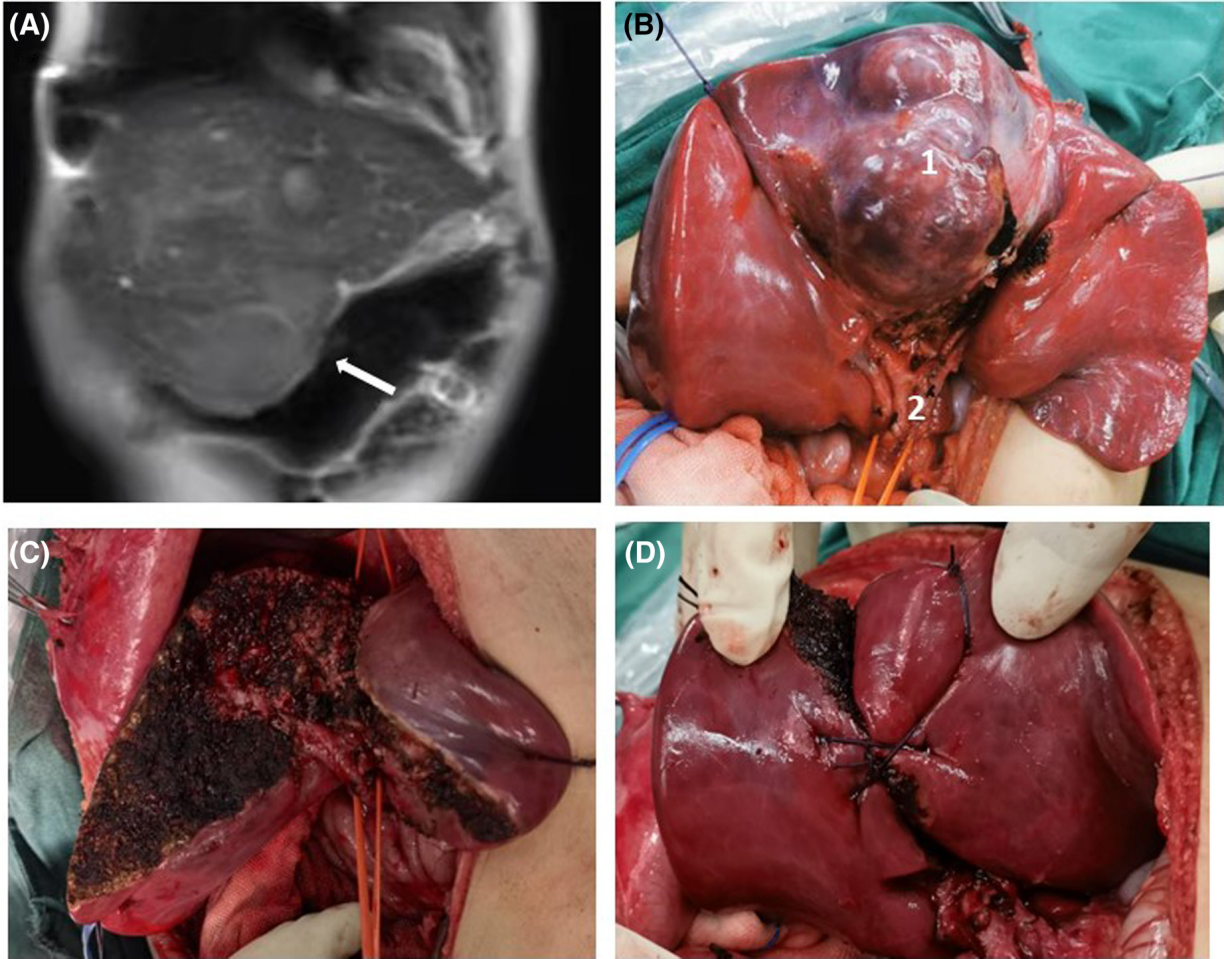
Middle‐lobe hepatectomy on a 9‐month‐old patient with central hepatoblastoma: (A) Magnetic resonance imaging revealed a central liver tumor in an infant. (B) 1 giant liver tumor, 2 anatomy of the first hepatic hilum. (C) Complete resection of the liver tumor. (D) Reconstruction of remaining liver tissue.
